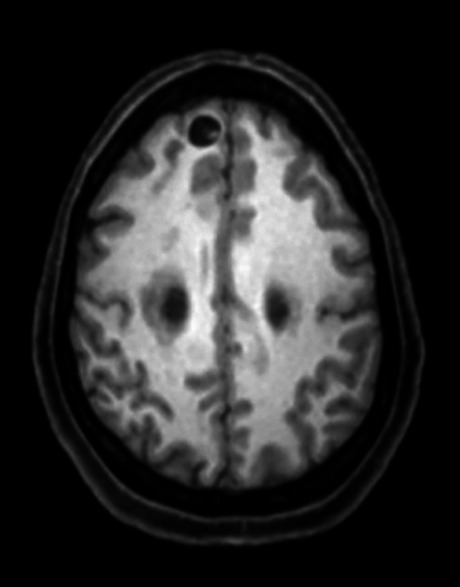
MRI intraparenchymal viable cysticercus with scolex.
